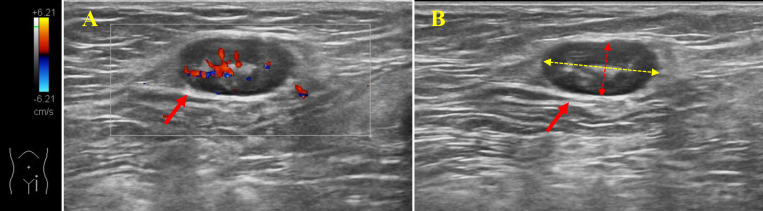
Ultrasonography of inguinal lymph nodes. (A): Color Doppler shows enlarged blood supply in lymph nodes in the inguinal region. (B): Measurements of the long and short axes of the lymph nodes. Solid red arrows: lymph nodes; Yellow dotted arrow: long axis of lymph node; Red dotted arrow: short axis of lymph node.
